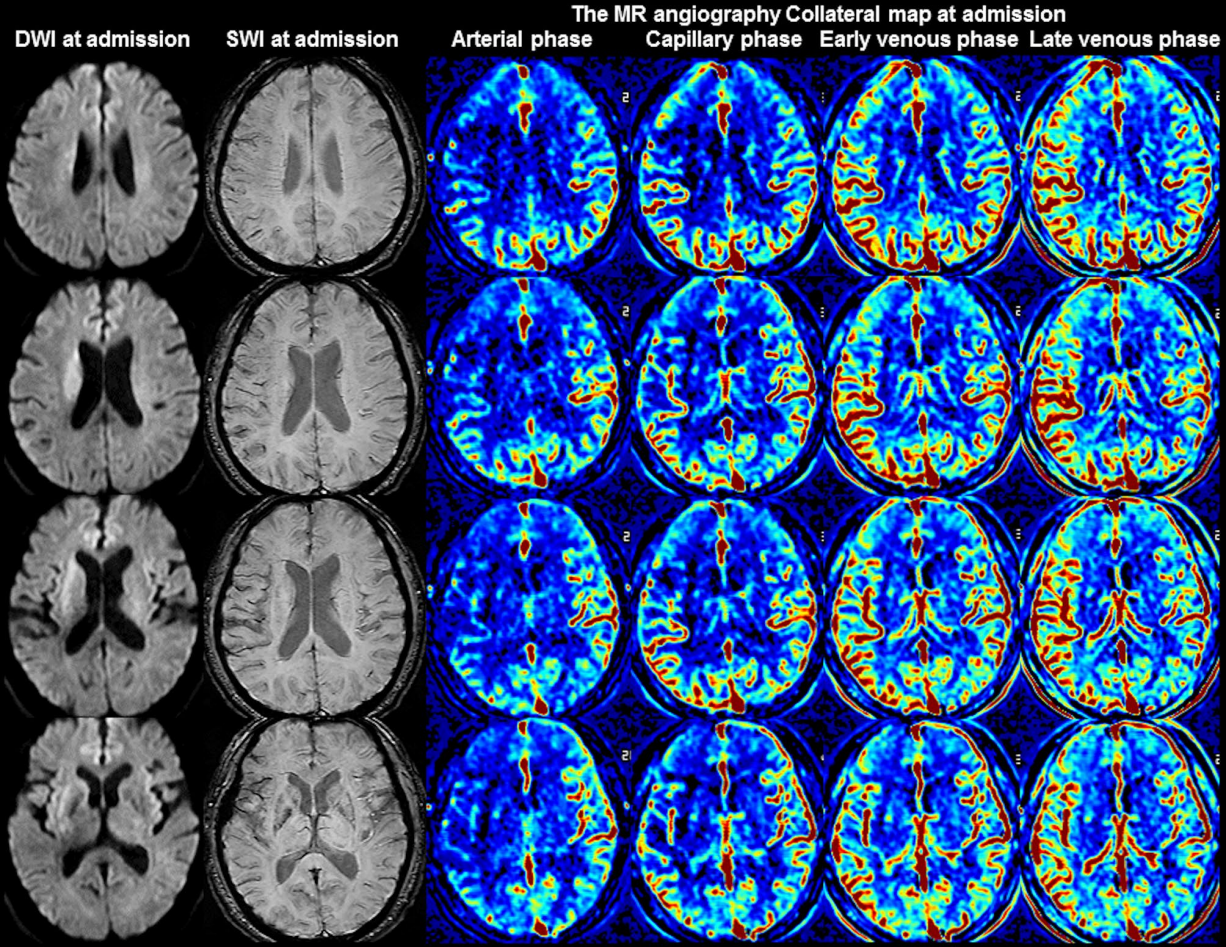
Images of a 62-year-old woman with occlusion of the right middle cerebral artery. The premorbid modified Rankin scale (mRS) score of this patient was 0, and the National Institutes of Health Stroke Scale score at admission was 8. The diffusion-weighted imaging (DWI), susceptibility-weighted imaging (SWI), and multiphase MR angiography collateral map at 1 h 40 min after symptom onset are shown. DWI shows acute infarct signals in the right basal ganglia, and SWI shows moderately prominent cortical and medullary veins in the right middle cerebral artery territory, representing poor collateral status. The MR angiography collateral map shows collateral-perfusion delay of more than one-half of the right middle cerebral artery territory in the capillary phase and less than one-half of the territory in the early venous phase (MR acute ischemic stroke collateral score of 2: intermediate to poor collateral perfusion defined as collateral-perfusion delay more than one-half of the ischemic MCA territory in the capillary phase and equal to or less than one-half in the early venous phase). The patient underwent intravenous thrombolysis followed by intraarterial thrombectomy, and the occluded arteries were completely recanalized at 2 h 45 min after symptom onset. The patient recovered, as shown by the 90-day mRS score of 1.
